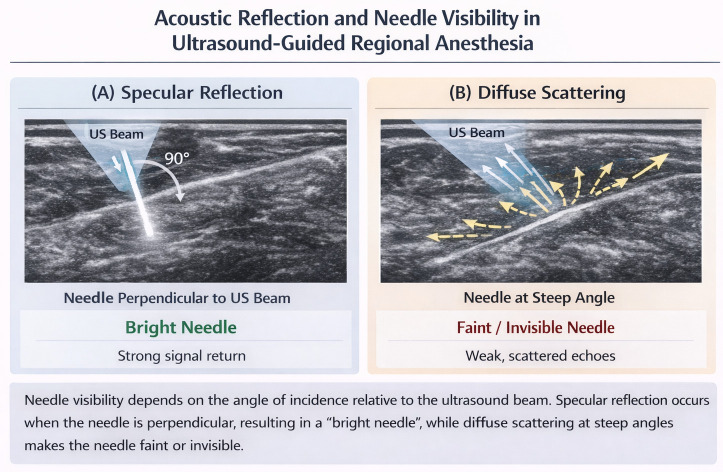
Acoustic reflection mechanisms and their impact on needle visibility during ultrasound-guided regional anesthesia.
